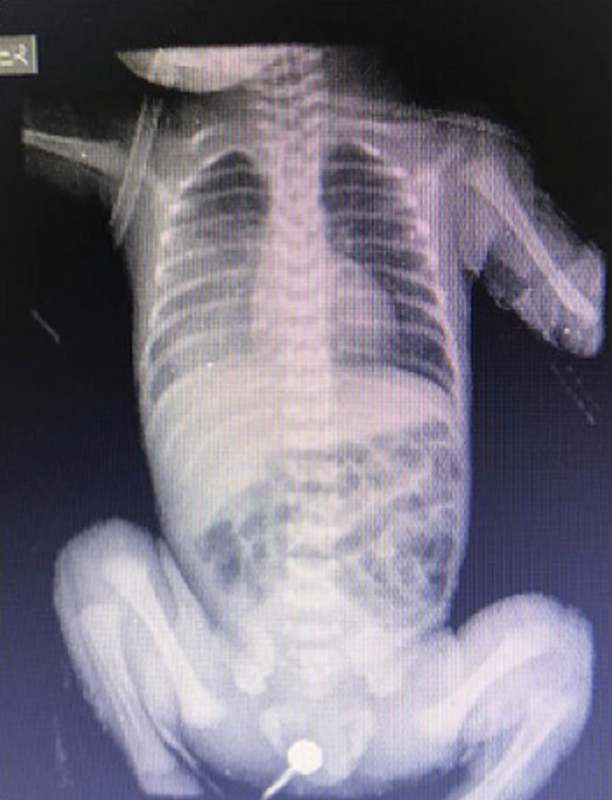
Full body X-rays showing opacities in the right hemithorax and air bronchogram.
